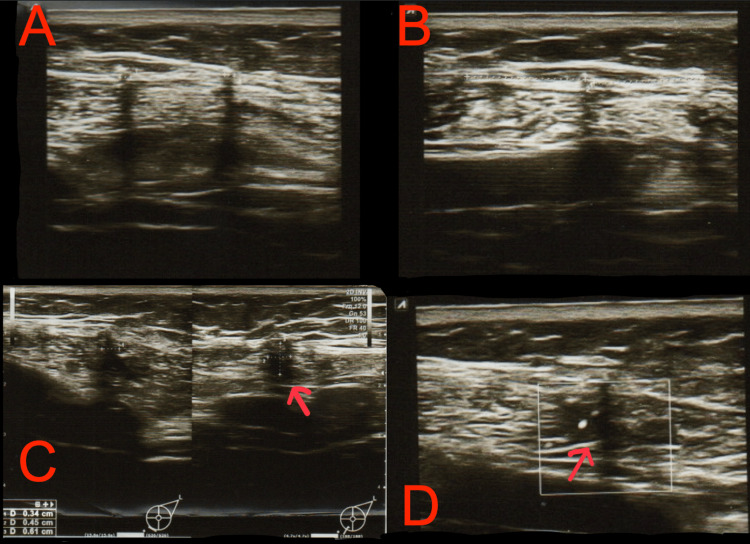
Breast ultrasound examination on January 23, 2023 A: Layered structure of the chest wall tissues after mastectomy with smooth contour of the tissue expander; B: Layered structure of the chest wall tissues after mastectomy with smooth contour of the tissue expander; C: Hypoechoic area measuring 3×5×6 mm with single vascular flows (red arrow); D: Single vascular flows within the 3×5×6 mm lesion (red arrow).
